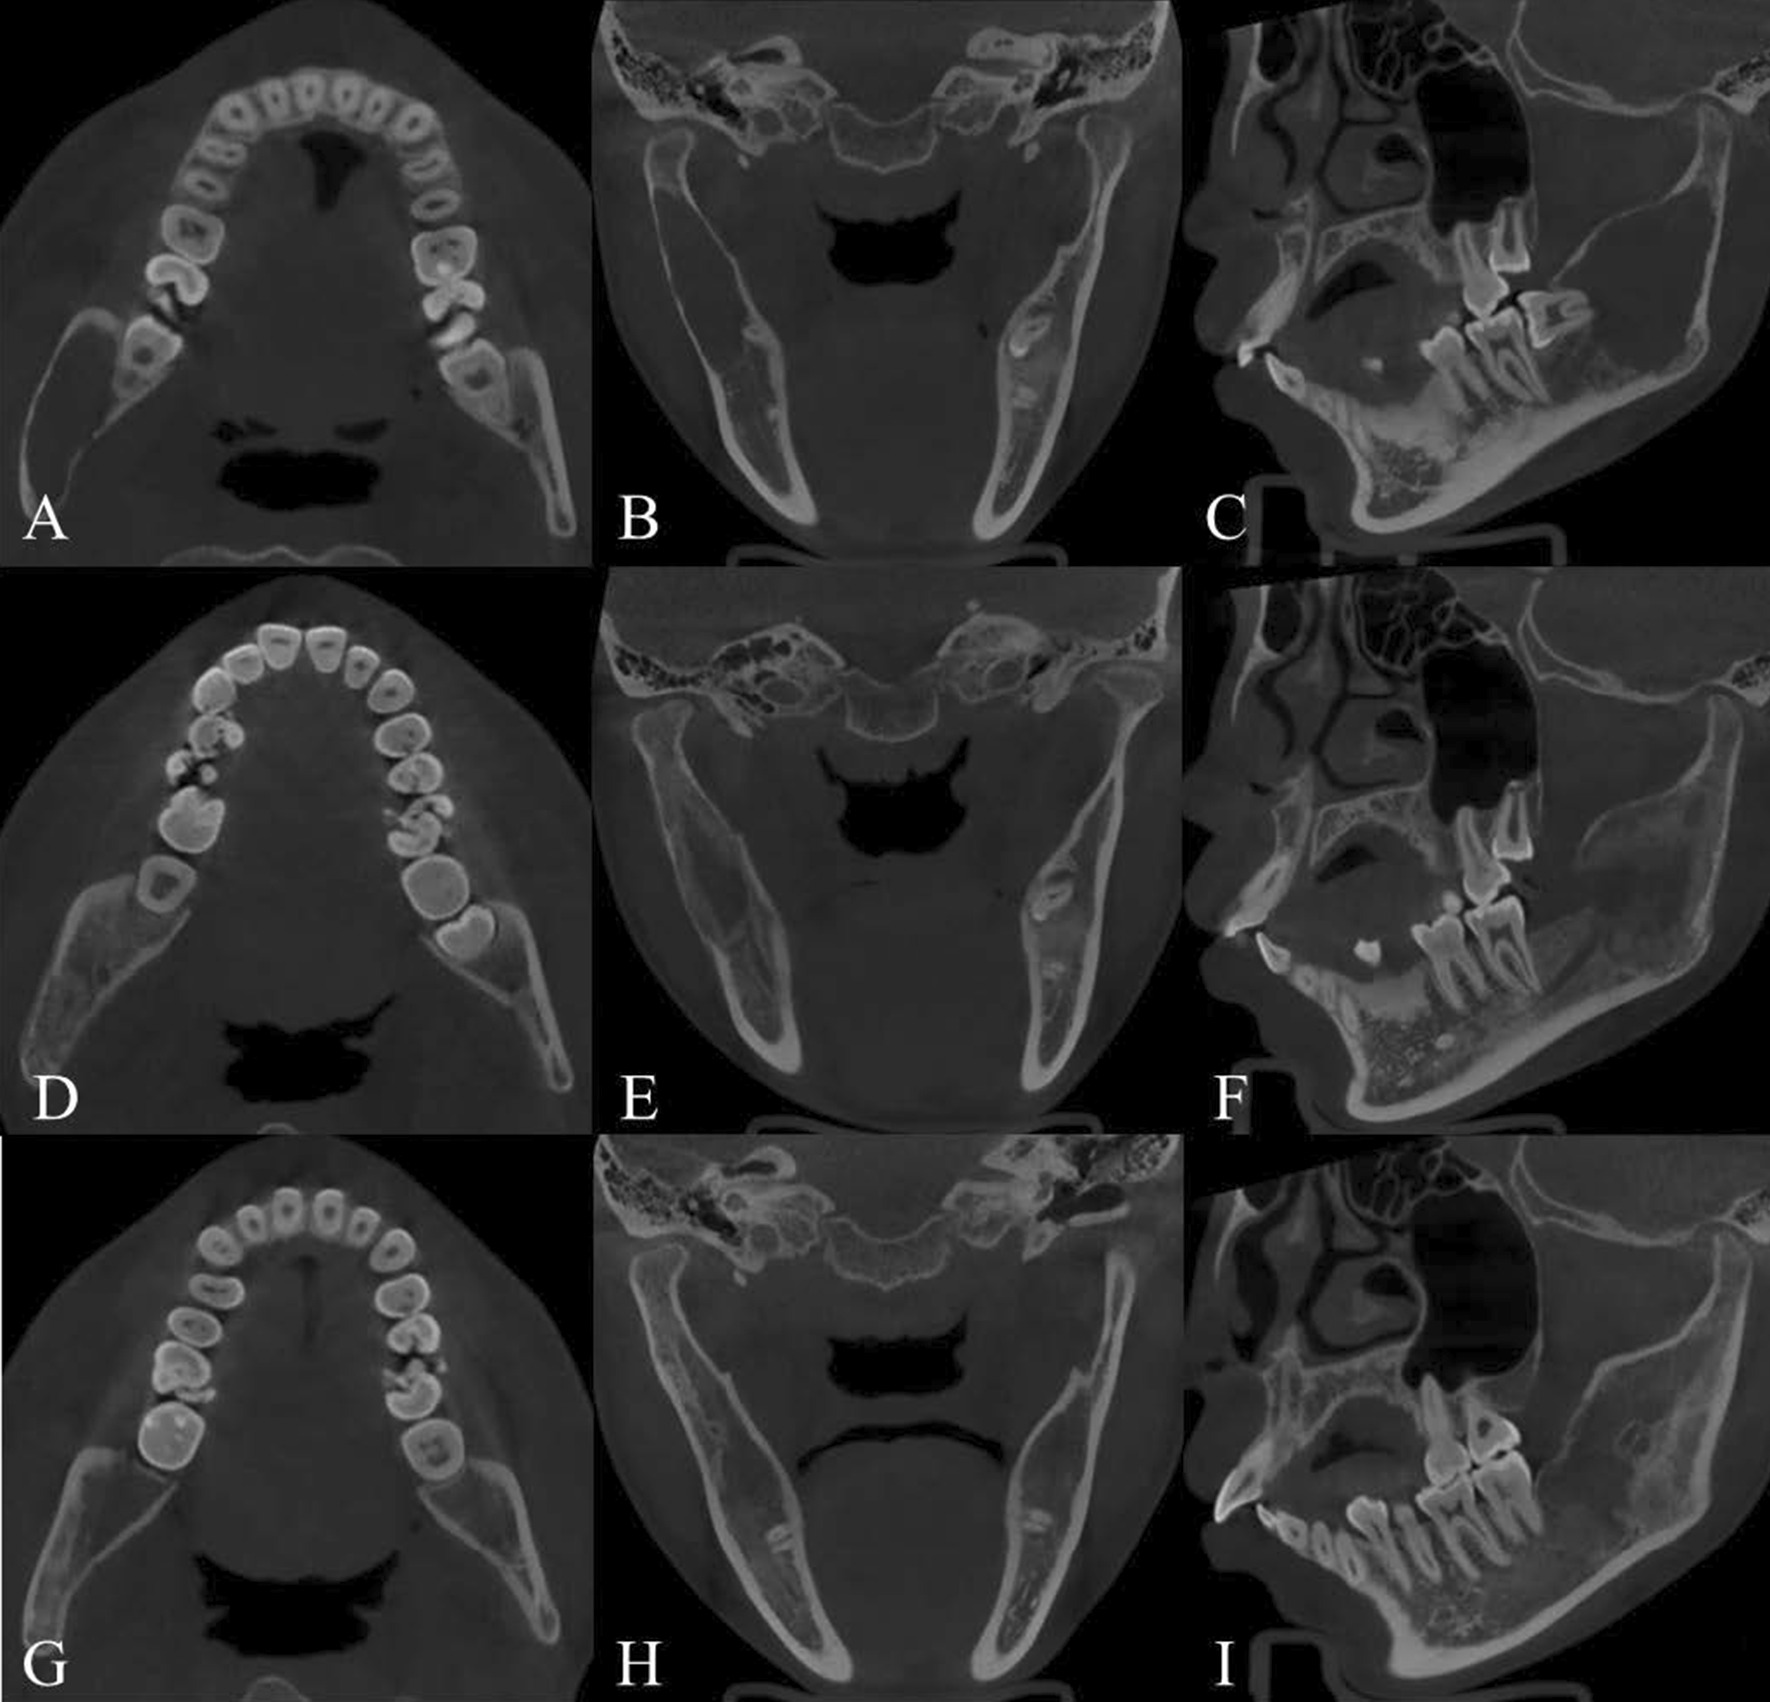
Radiographic features of ABC. A–C CBCT preoperatively for ABC. CBCT shows a large irregular, unilocular radiolucent image of the right mandibular body and ramus with thinning of the buccal and lingual cortex without bone-white lines and no significant resorption or displacement of the adjacent roots. D–F CBCT at 3 months postoperation of ABC. CBCT shows osteogenesis in the right mandibular body and ramus and thickening of the buccal and lingual cortex compared to the preoperative period. G–I CBCT 6 months postoperation of ABC. CBCT shows further osteogenesis in the right mandibular body and ramus with higher density than three months earlier and further thickening of the buccal and lingual cortex compared to the 3 months postoperation
